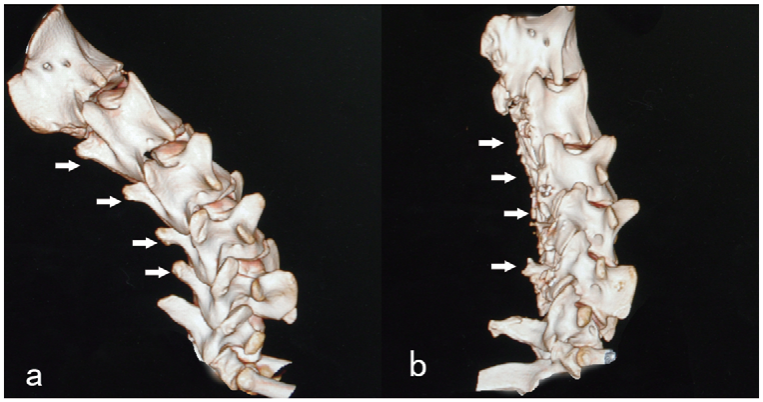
CT reconstruction of the cervical spine. a show the preoperative picture of the spinous process (C3–C6, arrows); b show the picture of the spinous process six months after surgery, the spinous process of the surgical vertebra is almost gone (C3–C6, arrows).
